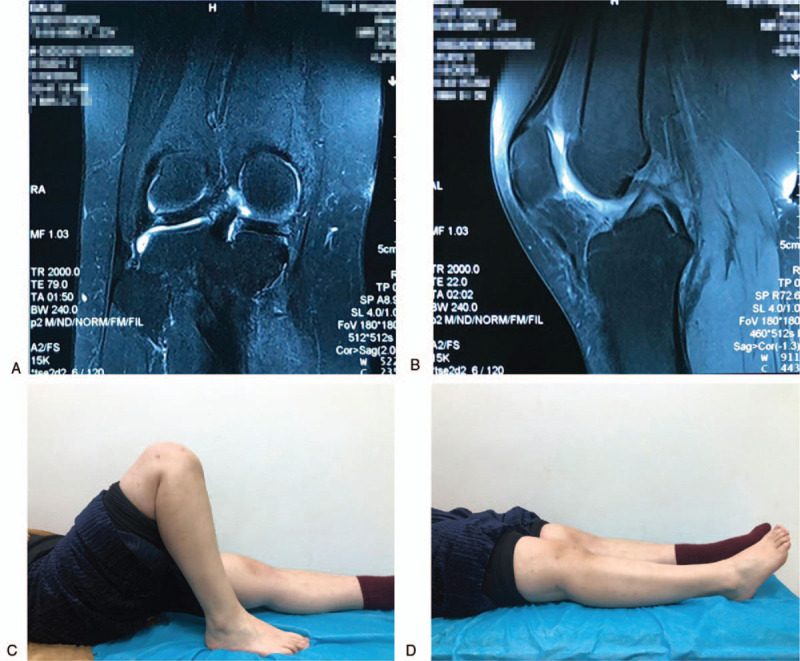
Follow-up MRI performed 6 mo after the second surgery. (A) Sagittal and (B) coronal MRI T2WI sequences showed that there was no recurrence. (Patient information for this figure has been blocked intentionally). (C, D) Postoperative assessment found that the range of motion of the right knee was normal. MRI = magnetic resonance imaging.
